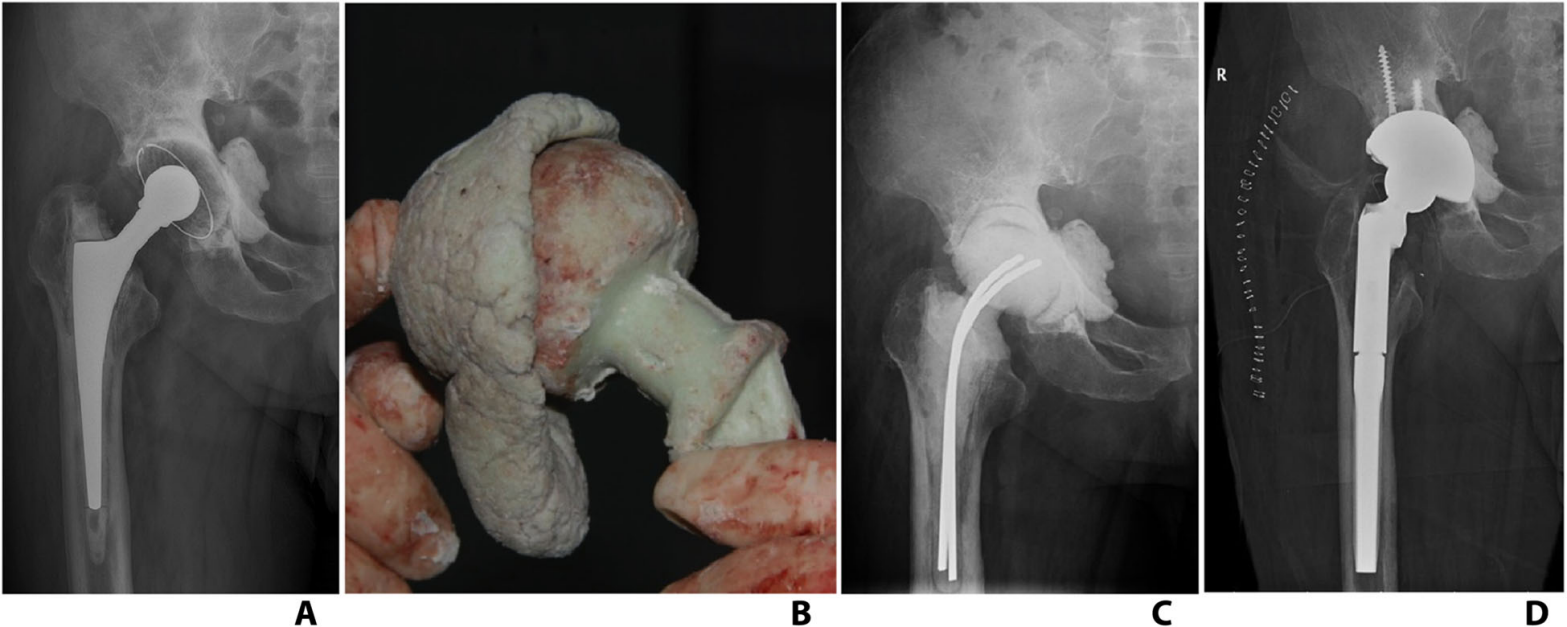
a One case with Paprosky IIC acetabular bone defect. b Handmade acetabular spacer was applied to reconstruct medial wall. d X-ray after spacer implantation. f X-ray after prosthesis implantation
